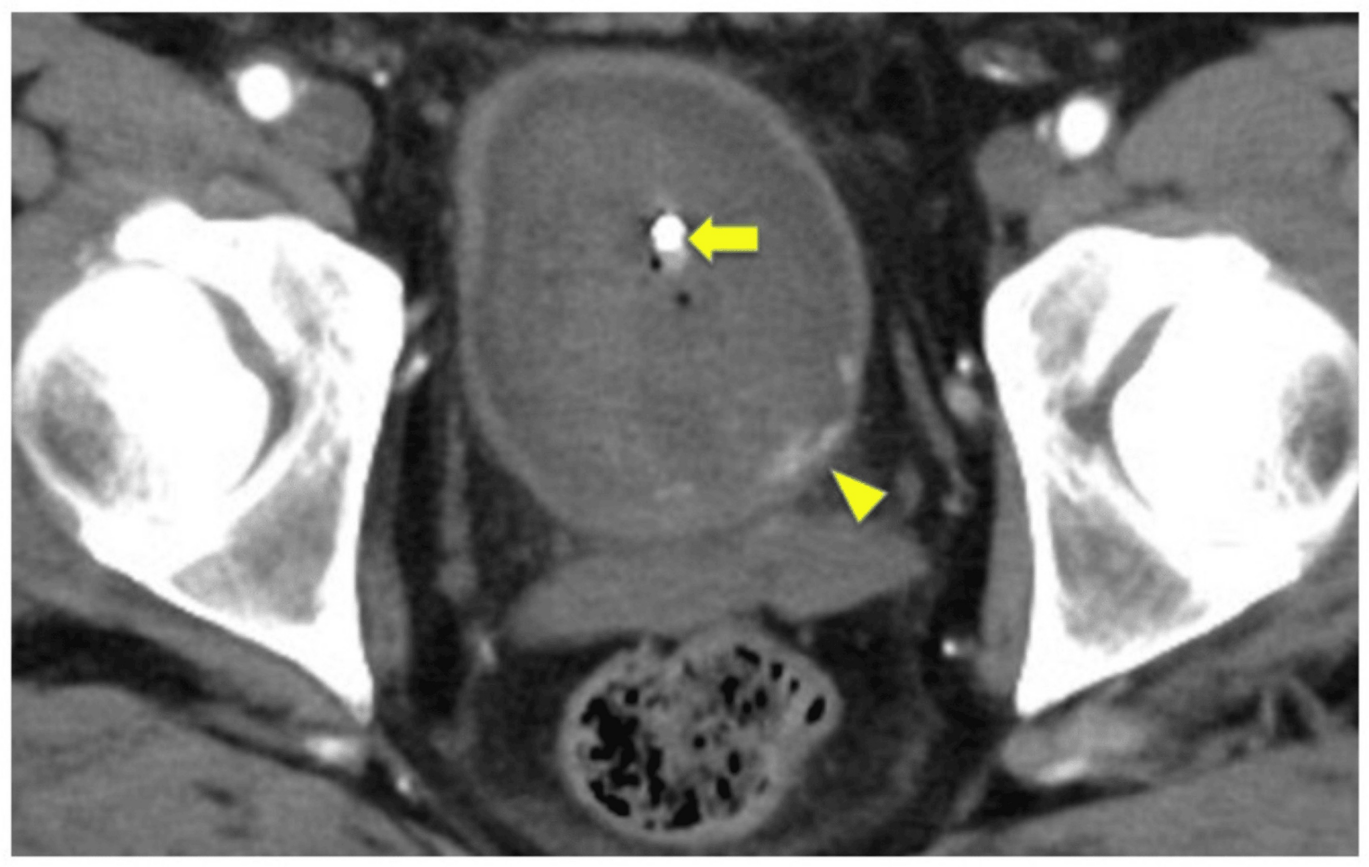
Contrast-enhanced pelvic CT (arterial phase). Contrast extravasation (arrowhead) is seen from the bladder neck at the five o’clock position; the catheter is indicated by the arrow.
